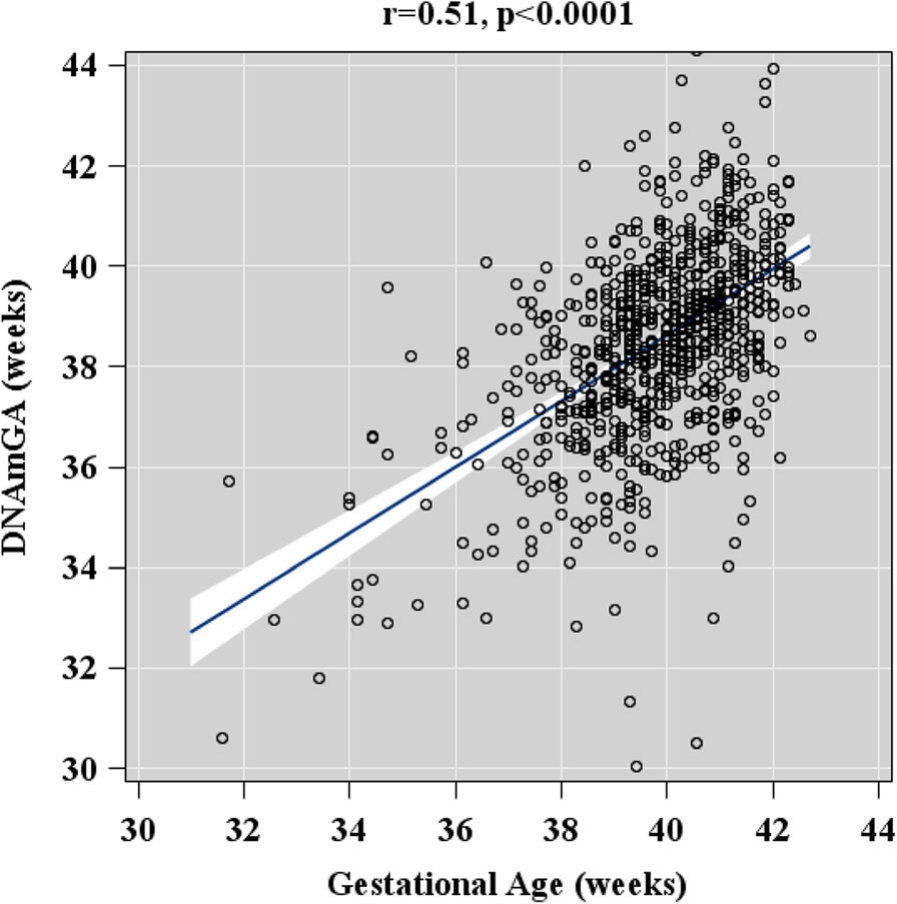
Association between offspring DNAm gestational age (GA) at birth based on cord blood methylation data and offspring chronological ultrasound-based GA at birth. *Scatterplot* shows regression line and 95% confidence intervals. *p* value refers to the significance level of the association
